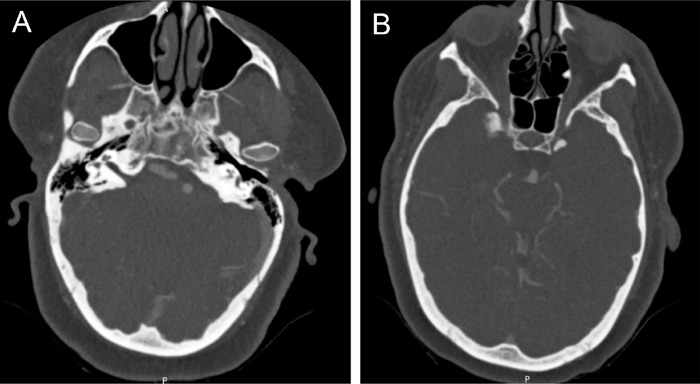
(A) Axial computed tomography angiography (CTA) image of the skull base shows the absence of petrous carotid canals. (B) Axial CTA image of the brain demonstrates absent intracranial carotid flow above the cavernous sinuses.
